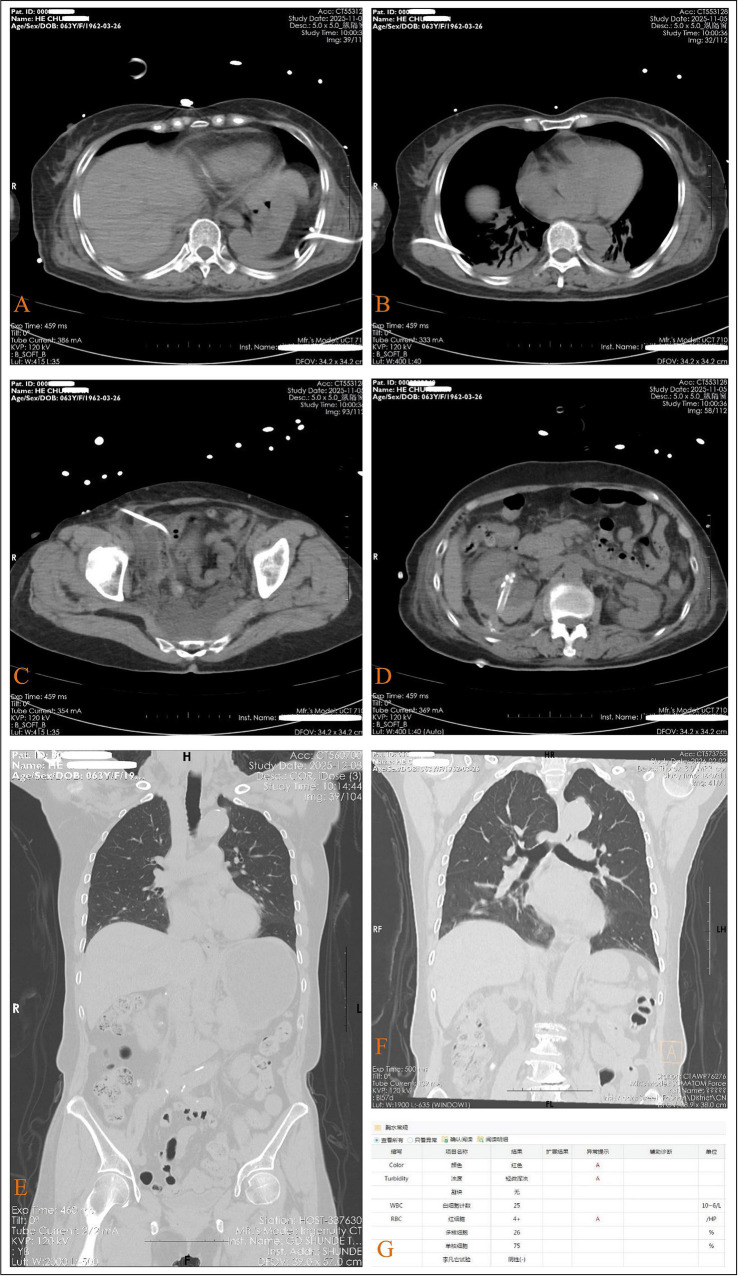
Postoperative chest and abdominal drains, follow-up CT imaging, and hemorrhagic pleural fluid analysis. (A) Left chest: chest tube *in situ*. (B) Right chest: chest tube in situ. (C) Abdomen: abdominal drain in situ. (D) Right kidney: nephrostomy tube in place. (E) One-month follow-up thoracoabdominal CT: no abnormalities. (F) Three-month follow-up thoracoabdominal CT: unremarkable. (G) Postoperative pleural fluid analysis: red blood cell count 4 + per high power field. All image captures preserved original metadata including essential information and dates, while patient names and institutional details were redacted in compliance with privacy protection protocols.
